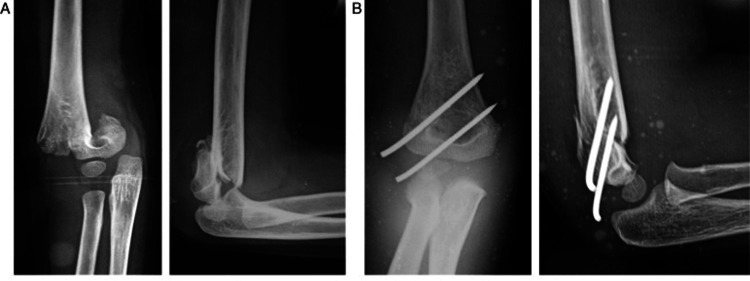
Parallel lateral K wires A: Preoperative anteroposterior and lateral radiographs of an eight-year-old boy; B: Immediate postoperative radiograph with good reduction
